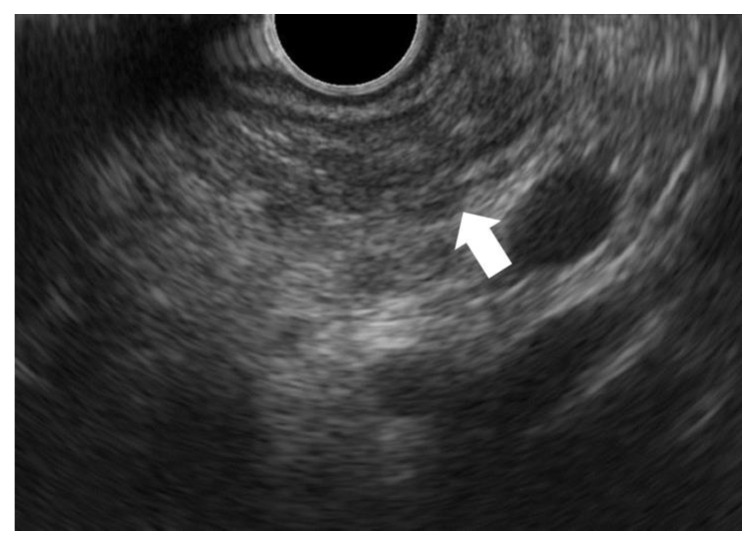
Endoscopic ultrasonography (EUS) finding of focal pancreatic parenchymal atrophy (FPPA). FPPA can be observed on EUS as a blurred hypoechoic area (arrow).
